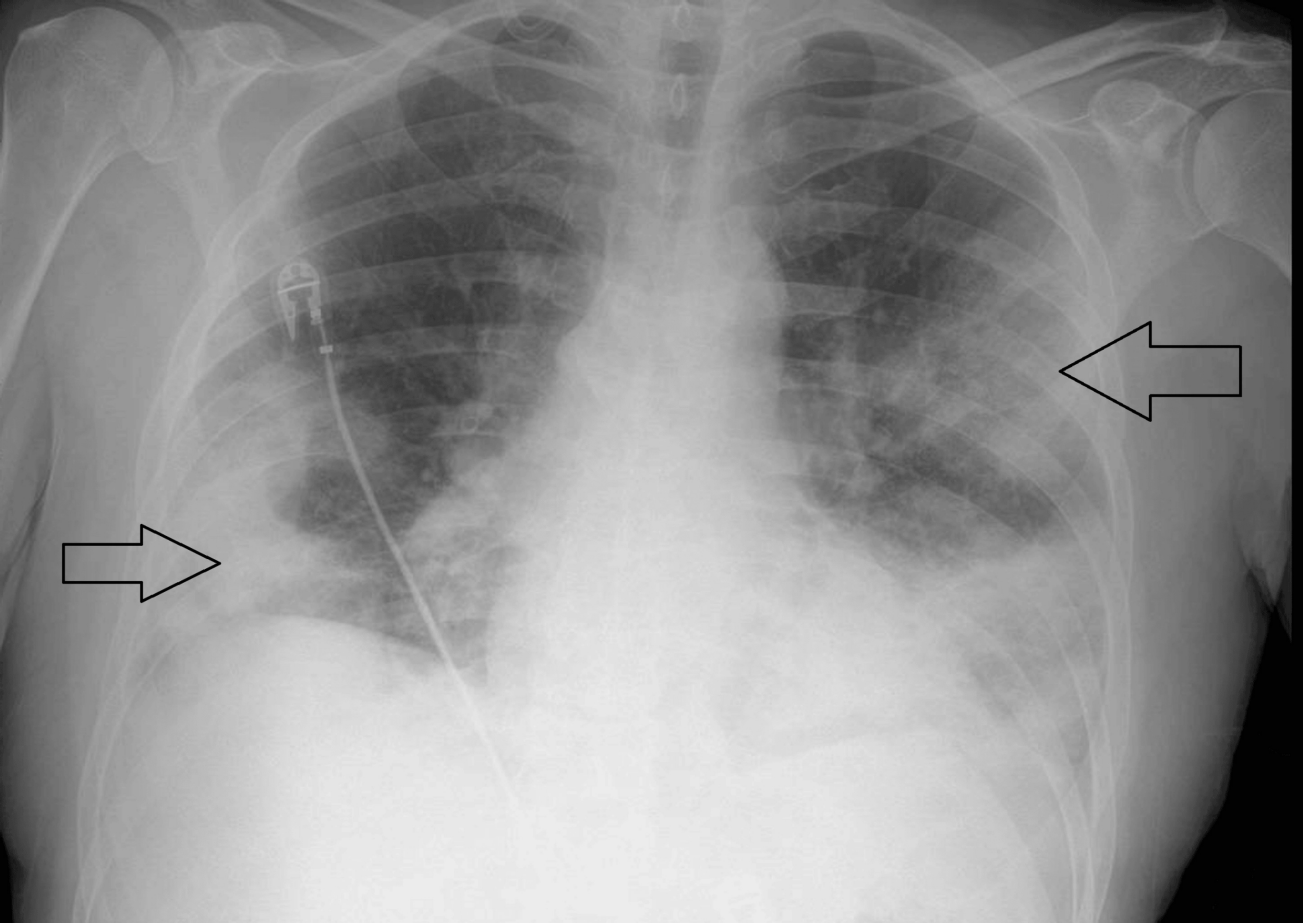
Chest X-ray showing bilateral patchy consolidation.
